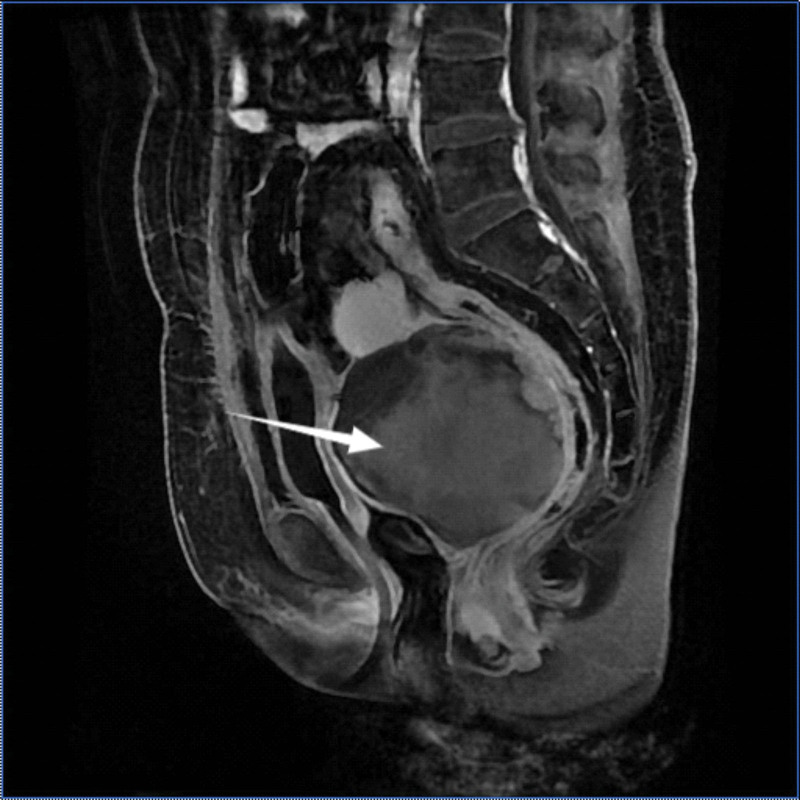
Pelvic MRI plain scan + enhanced: cystic and solid mass in the vaginal rectal space of some 11 cm × 10 cm × 14 cm in size; the signal was not uniform, the edge of TIWI was mainly iso-low signal, and patchy high signal could be seen in it. T2WI and T2 lipid-suppressed edge showed high signal, and iso- and slightly high signal were seen in them. MRI = magnetic resonance imaging.
